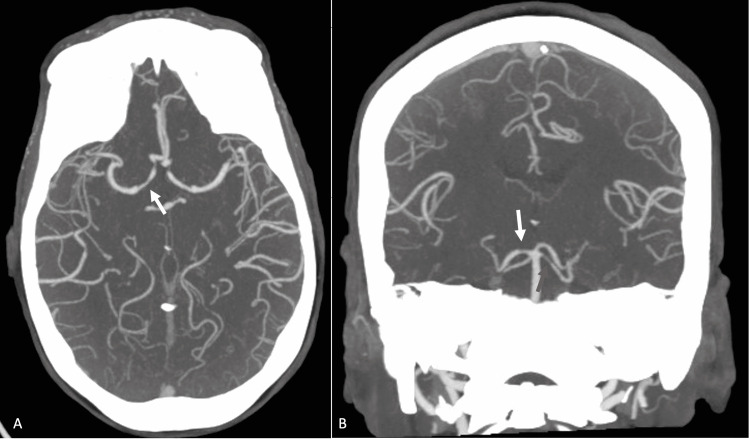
Axial and coronal MIP post-contrast CTA image of the head A: Axial MIP post-contrast CTA image of the head demonstrates a focal narrowing of the A1 segment of the right ACA (white arrow). B: Coronal MIP post-contrast CTA image of the head demonstrates focal narrowing of the P1 segment of the right PCA (white arrow) and focal narrowing of the proximal left superior cerebellar artery (red arrow).
